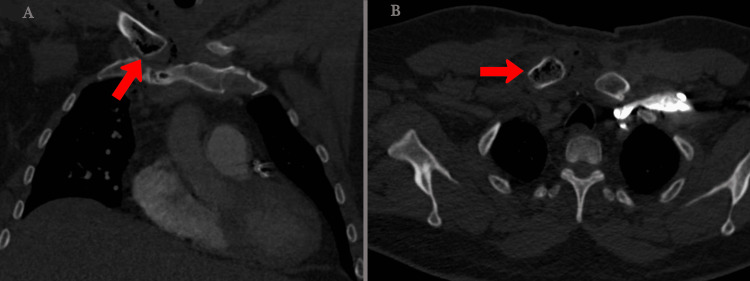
Computed tomography of the chest wall and bones with contrast. The images show (A) coronal cut of computed tomography and (B) axial cut of computed tomography showing right SCJ erosive changes, effusion, soft tissue thickening, and clavicle gas bubbles.
